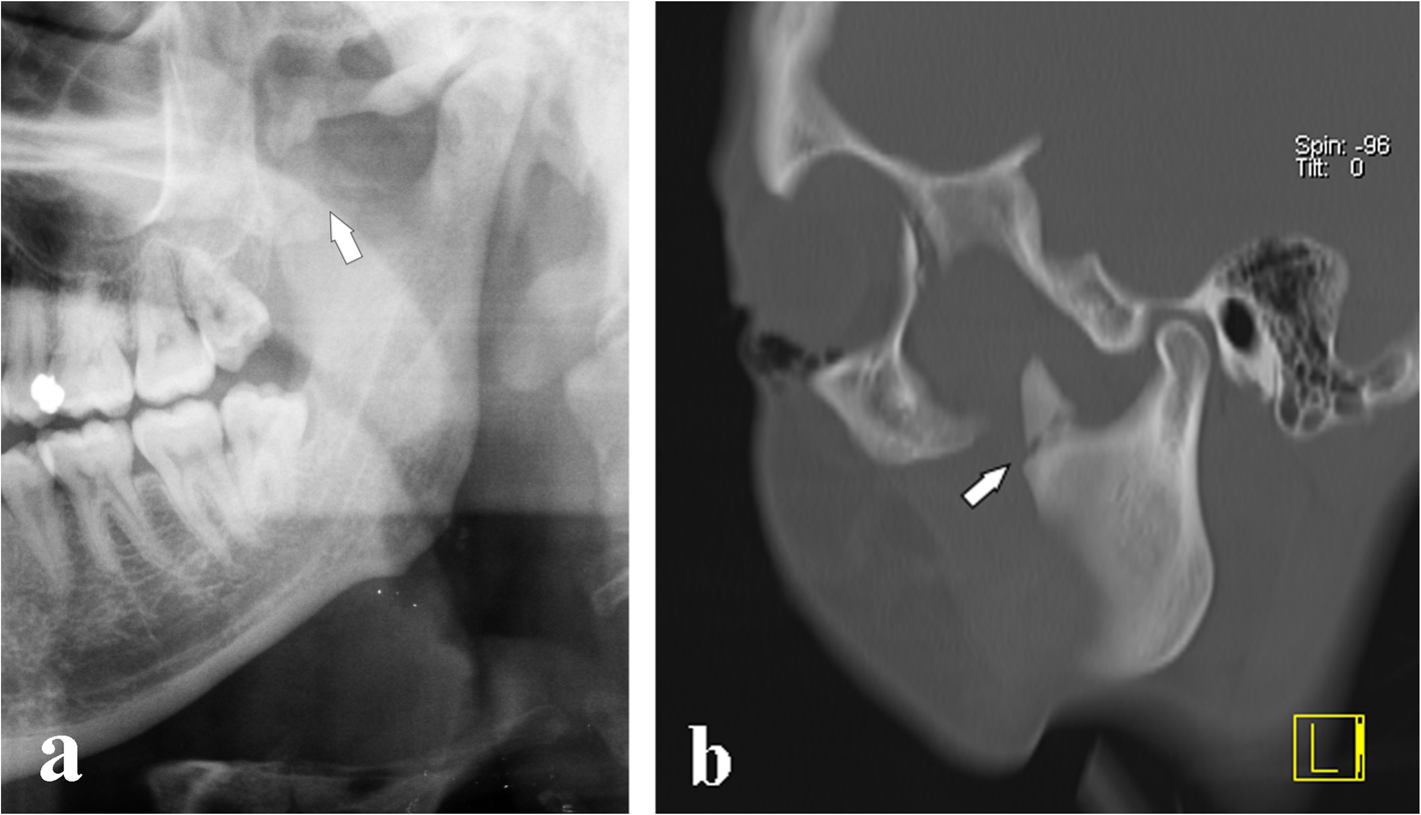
Coronoid process apex fracture. a Cropped panoramic radiograph. The fracture rhyme (arrow) runs from the internal to external faces of the coronoid process. b Computed tomography 5-mm oblique reconstruction of the same patient. Note that the fracture rhyme (arrow) ends higher than the sigmoid notch
